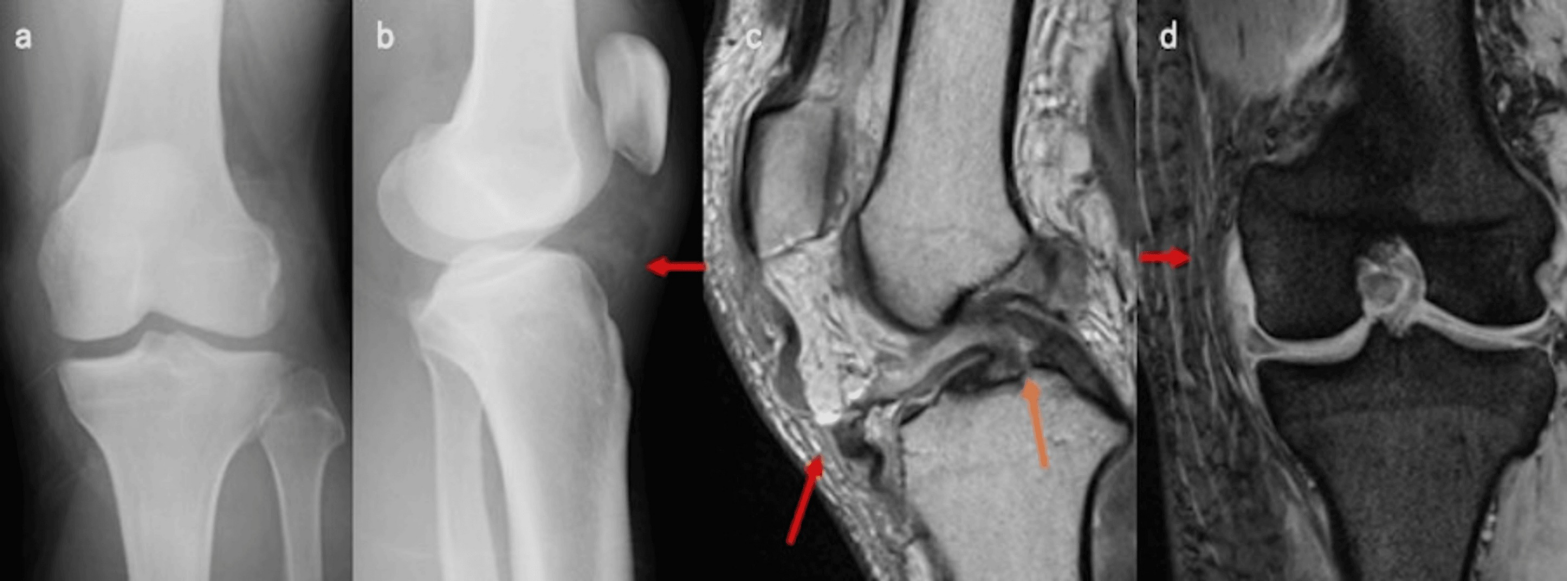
Preoperative radiograph and MRI findings (a, b) The Insall–Salvati ratio was 1.2. (c) MRI revealed a PT rupture at the mid-substance level (indicated by the red arrow) and an ACL rupture at the mid-portion (indicated by the orange arrow). (d) MCL rupture was observed at the femoral attachment site. MRI: magnetic resonance imaging, ACL: anterior cruciate ligament, MCL: medial collateral ligament, PT: patellar tendon
